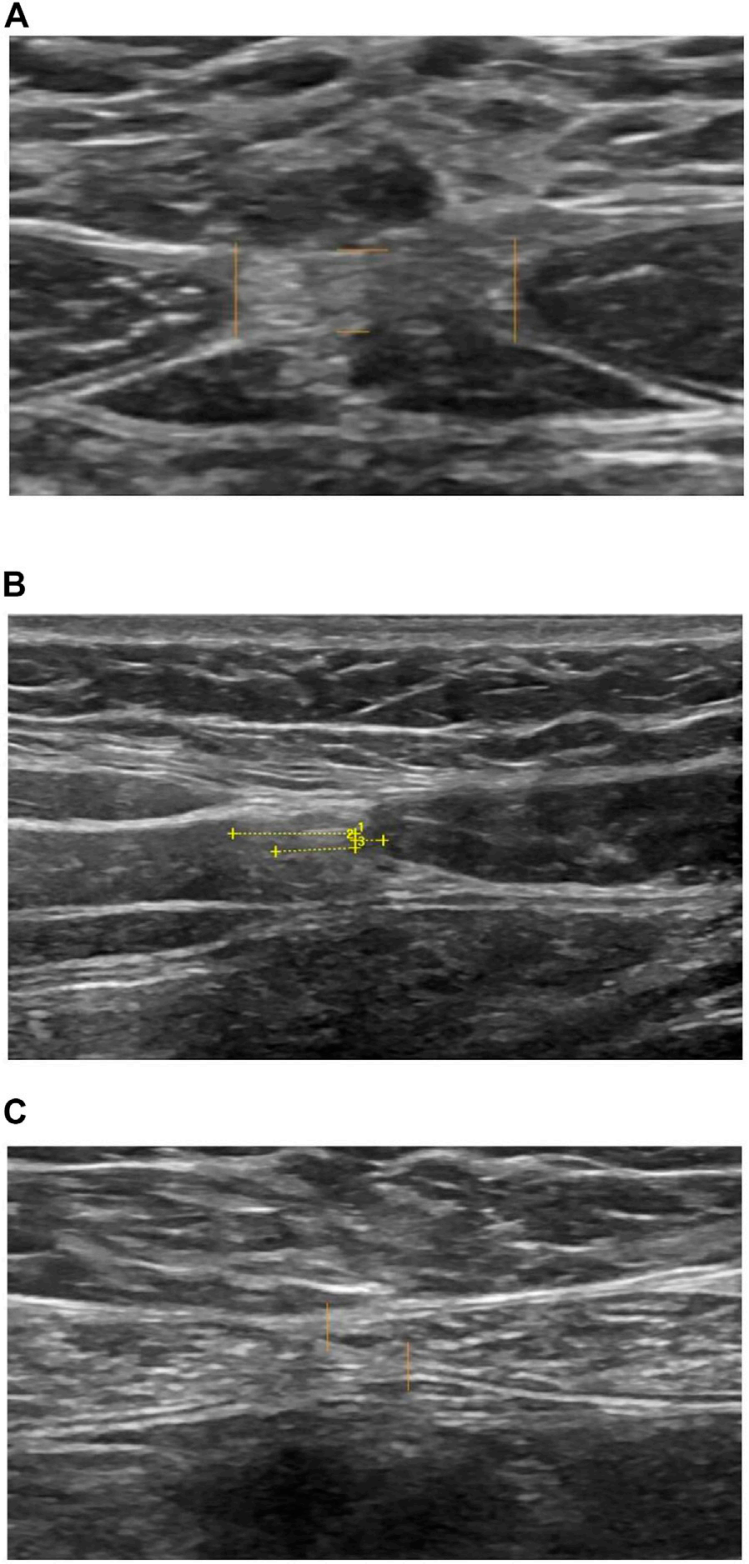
Ultrasound measurement. (A) Lina alba IRD and thickness. (B) Linea semilunaris at umbilical level. 1) Rectus abdominis muscle to external oblique muscle. 2) Rectus abdominis muscle to internal oblique muscle. 3) Rectus abdominis muscle to transverse abdominis muscle. (C) Linea alba 2 cm inferior to umbilicus, IRD negative value.
